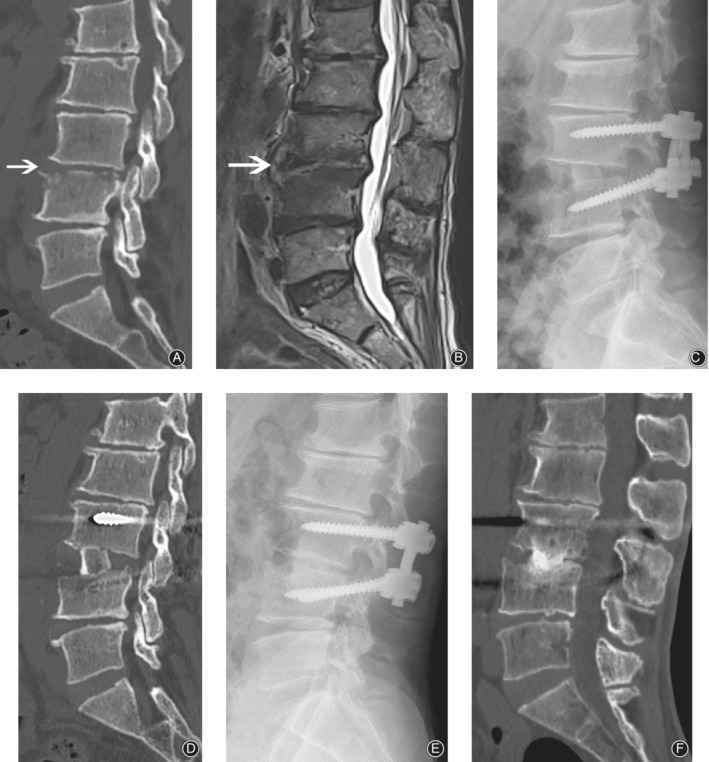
Fifty two‐year old man, with back pain for 2 months without fever. Pre‐operative CT scan (A) showed superior endplate destruction of L_4_. Pre‐operative MRI (B) shows high T2 signal in disc space and low T2 signal in both upper and lower endplate. Surgery was performed *via* as mentioned method. A massive structure bone graft was seen in the immediately postoperative film (C) and CT scan (D). One year after surgery, fusion was achieved between the interface in both film (E) and CT (F) scan. White arrow indicates the index level.
